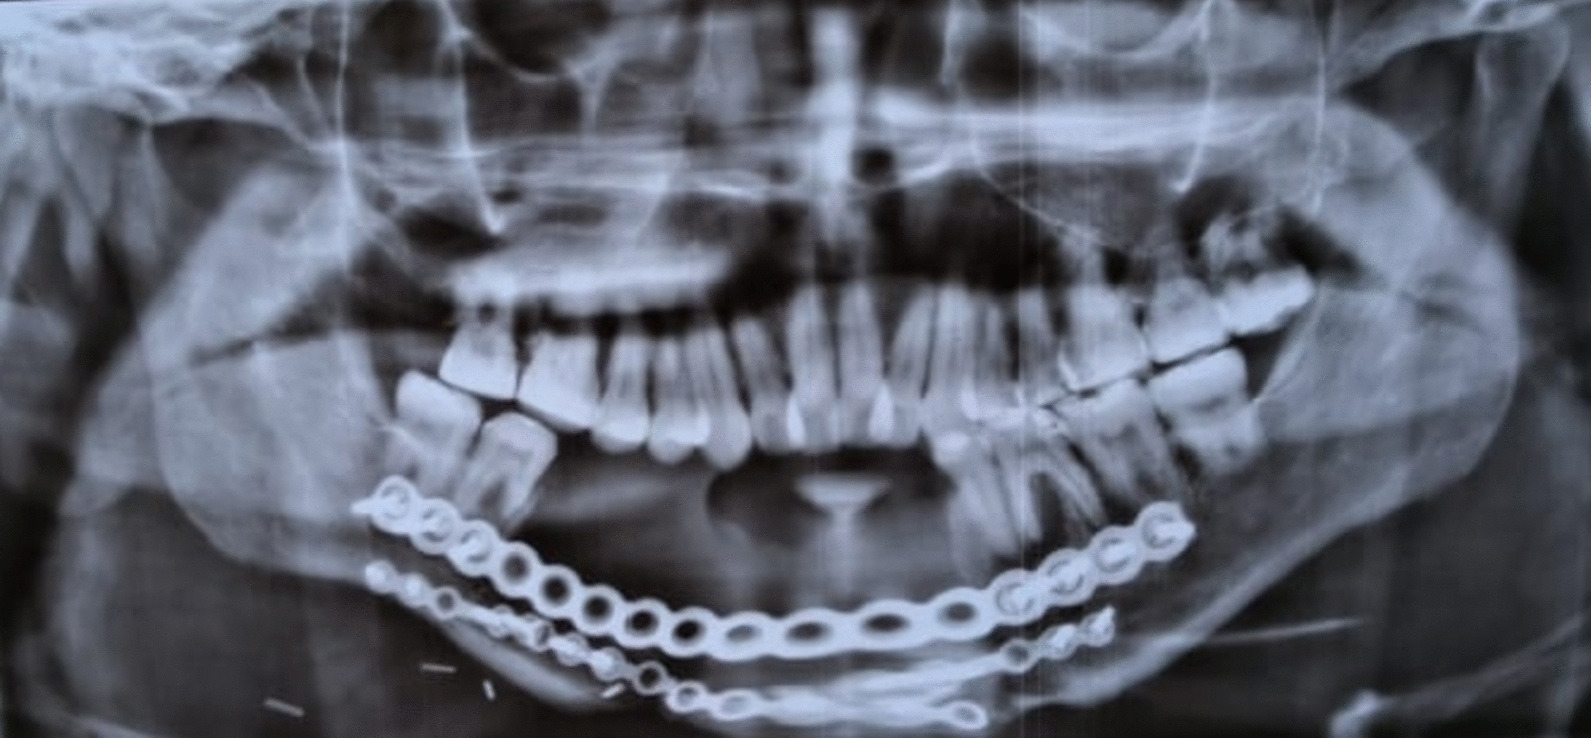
Orthopantomogram depicting segmental resection of anterior mandible reconstituted with reconstruction plates
